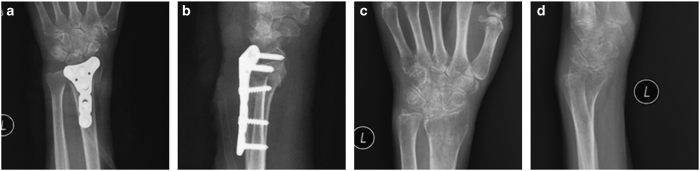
Radiographs of the man with a comminuted intra-articular fracture of the distal radius after treated by Dorsal locked plating. (a and b) Anteroposterior and lateral radiographs after one month, (c and d) Anteroposterior and lateral radiographs after one year.
